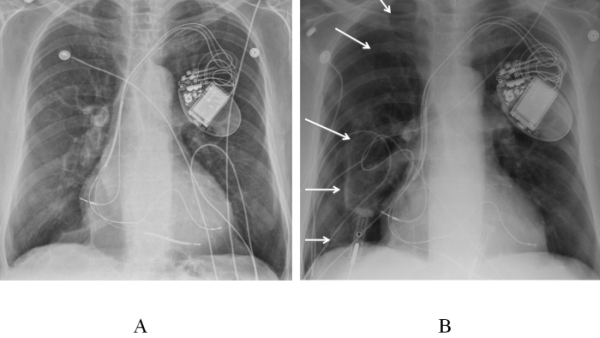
Chest radiographs after CRT system implantation (A) immediate post implant.(B)  Moderate sized Right pneumothorax (arrows)
